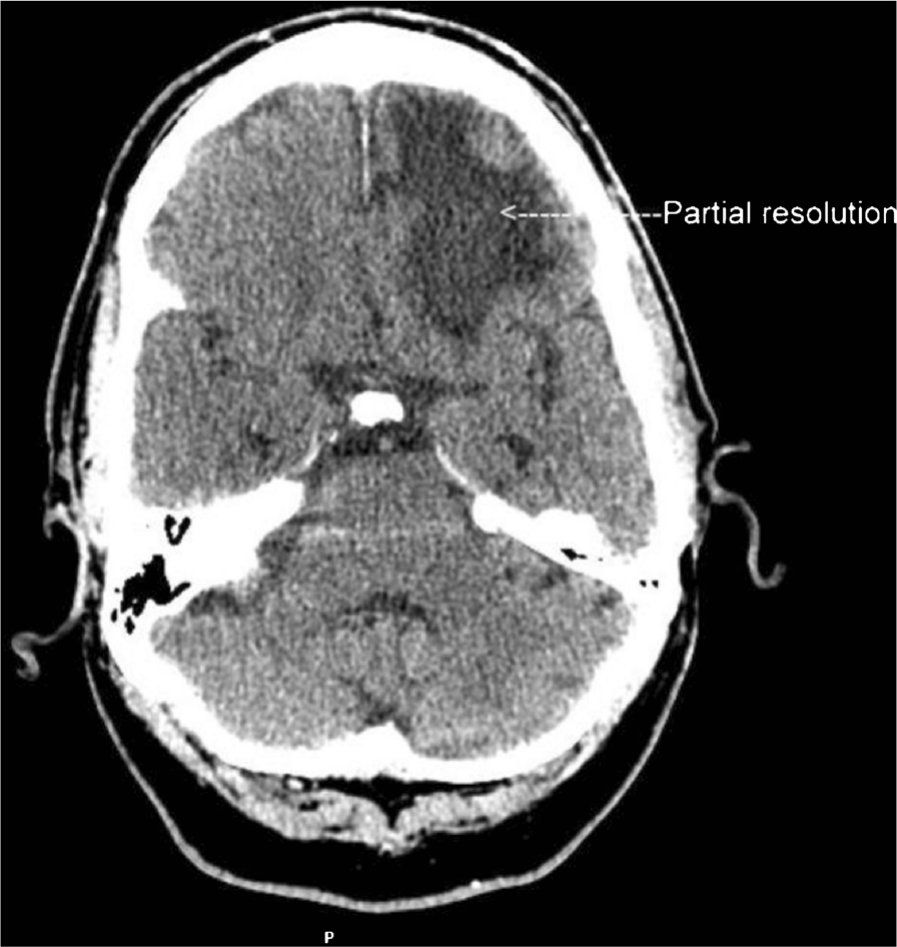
CT scan brain image of CASE 2 at 6 weeks of the presentation showing significant resolution of the hemorrhage with significant clinical improvement.
